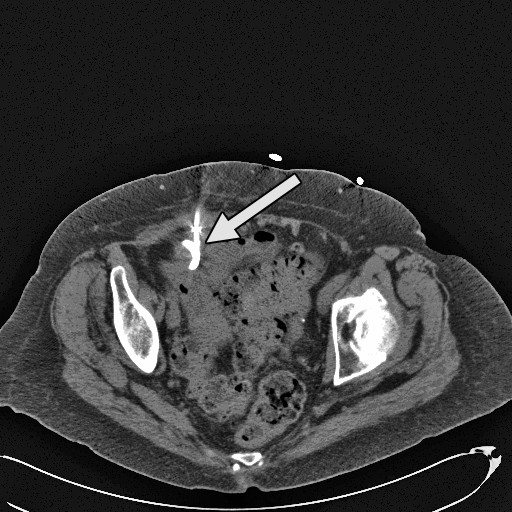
CT image of collapsed abscess cavity. CT image of the pelvis without contrast shows the drain in place and the abscess cavity completely collapsed.
